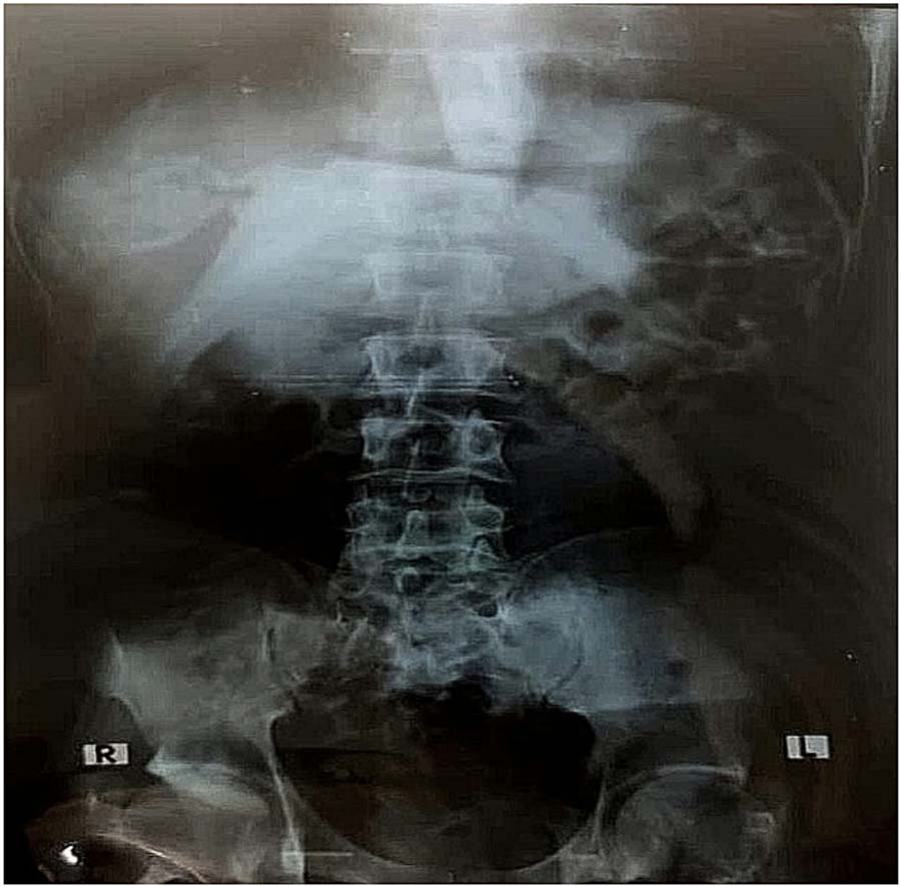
Abdominal X-ray showing no visible foreign body.
